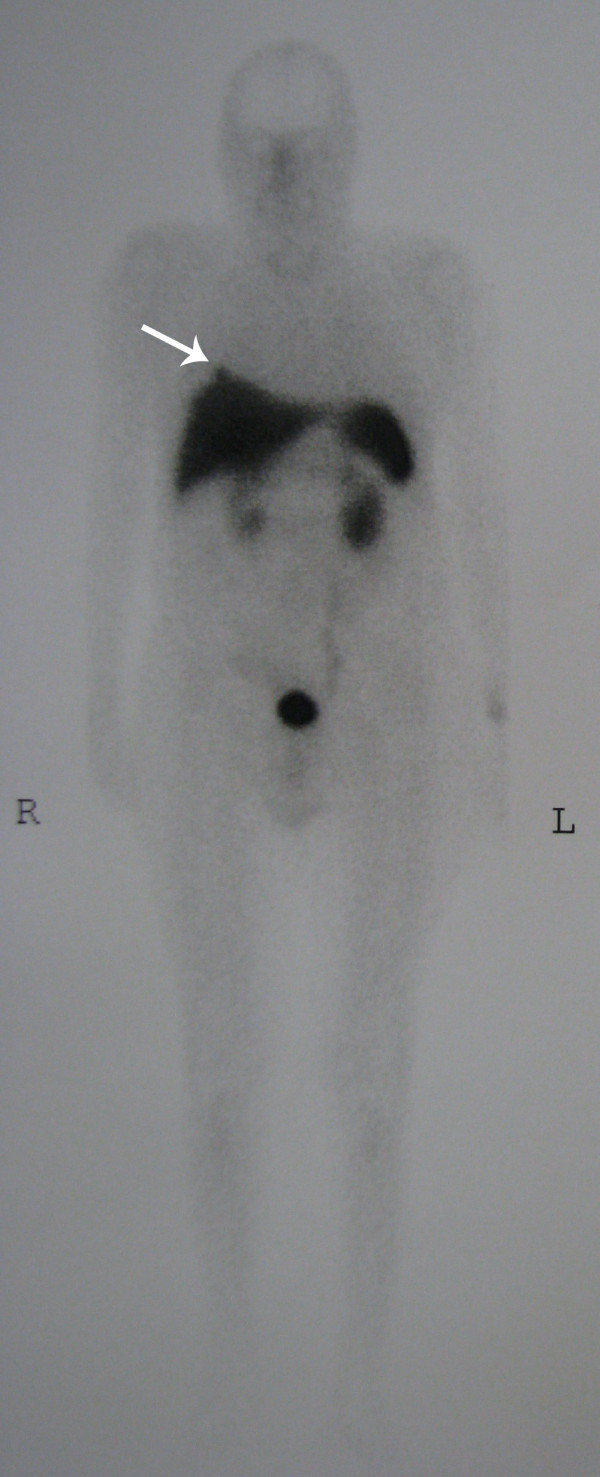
Technetium-99m-labeled octreotide acetate scintigraphy in the whole body planar view. This was performed 3 hours after injection of 15mCi technetium-99m-labeled octreotide acetate. There is a focal uptake in the lower lobe of our patient's right lung, just above his diaphragm, highly suggestive of an adrenocorticotropic hormone (ACTH)-producing bronchial tumor.
